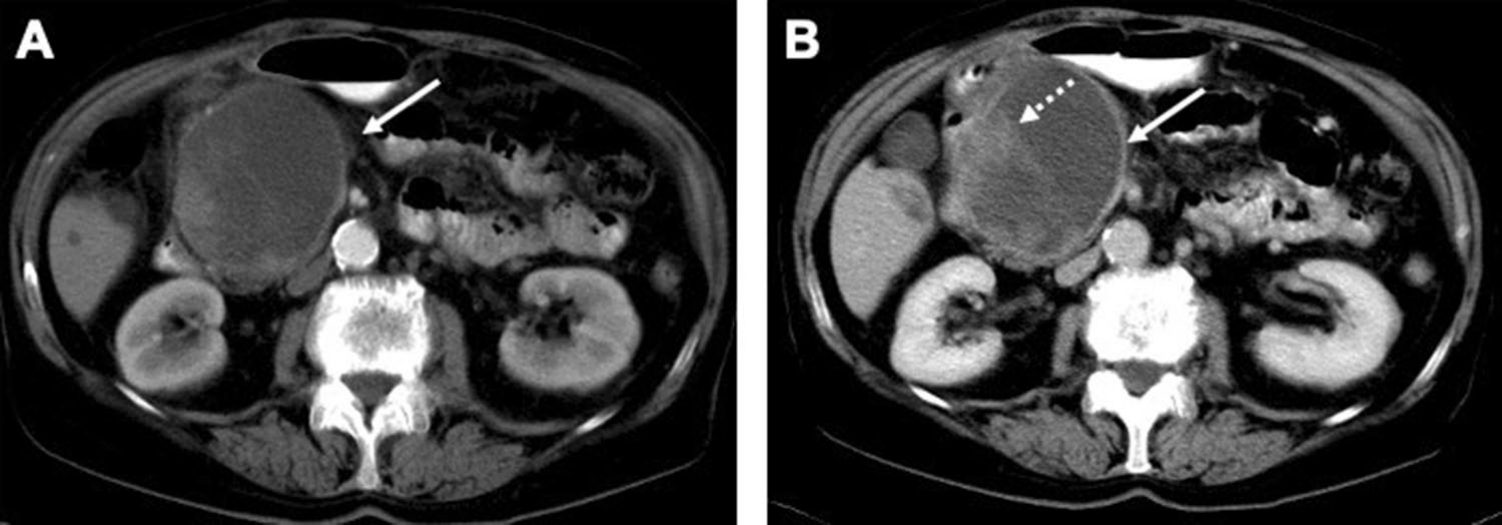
ITPN mimicking the morphology of mucinous cystic neoplasm. (A,B) Axial CT scans in the arterial phase (A) and portal venous phase (B) demonstration a huge oligocystic mass (arrow) in the pancreatic head with an enhancing solid nodular component (dotted arrow).
